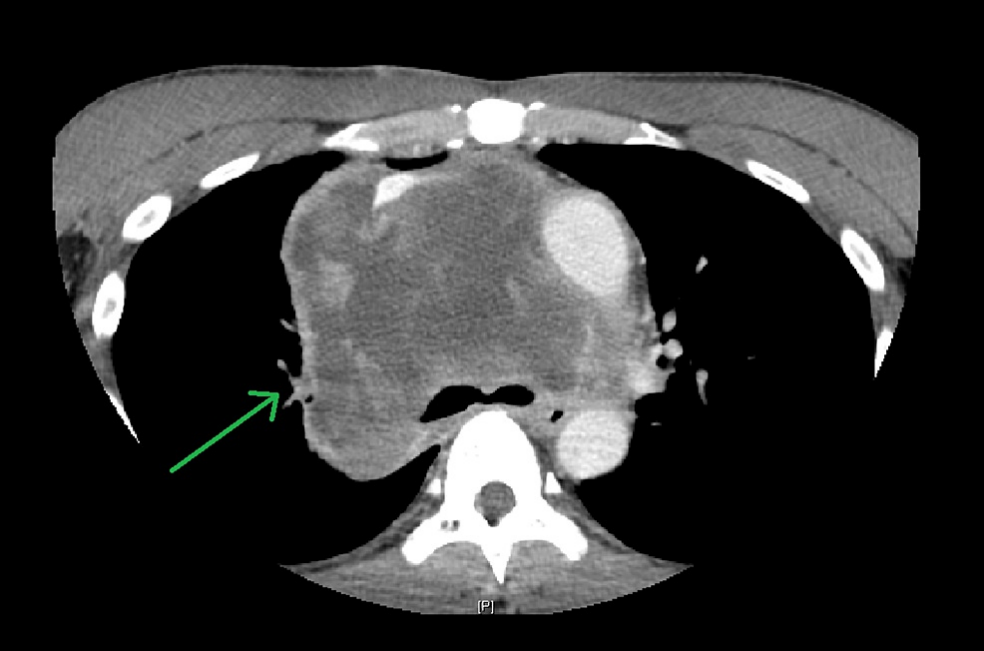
Computed tomography (CT) of the chest with intravenous contrast demonstrating the 13 cm x 10.5 cm x 11.2 cm heterogeneous mediastinal mass (green arrow) causing compression of the superior vena cava and right pulmonary artery and abutment of the adjacent vasculature
